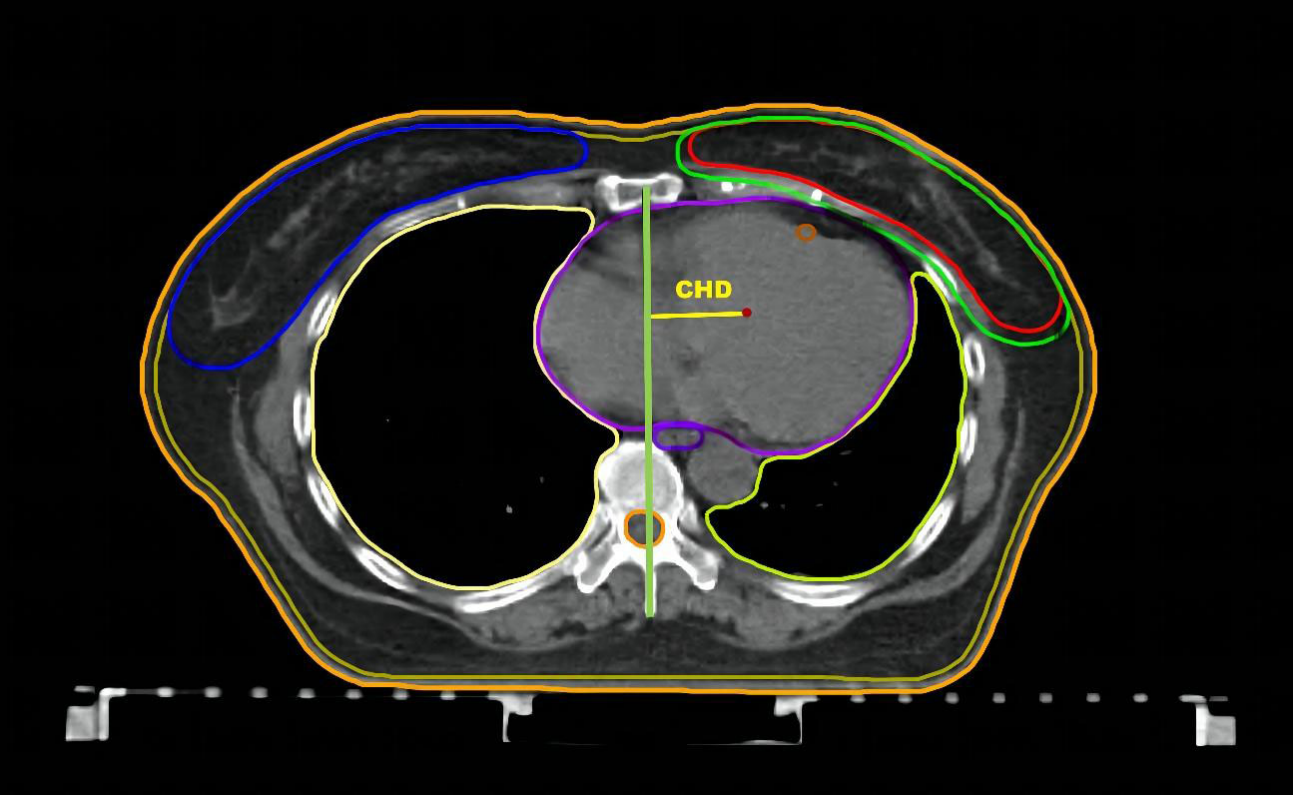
CHD in the supine CT. The central heart plane. The central heart distance (CHD) is the perpendicular distance from centre point of heart to the midline.
